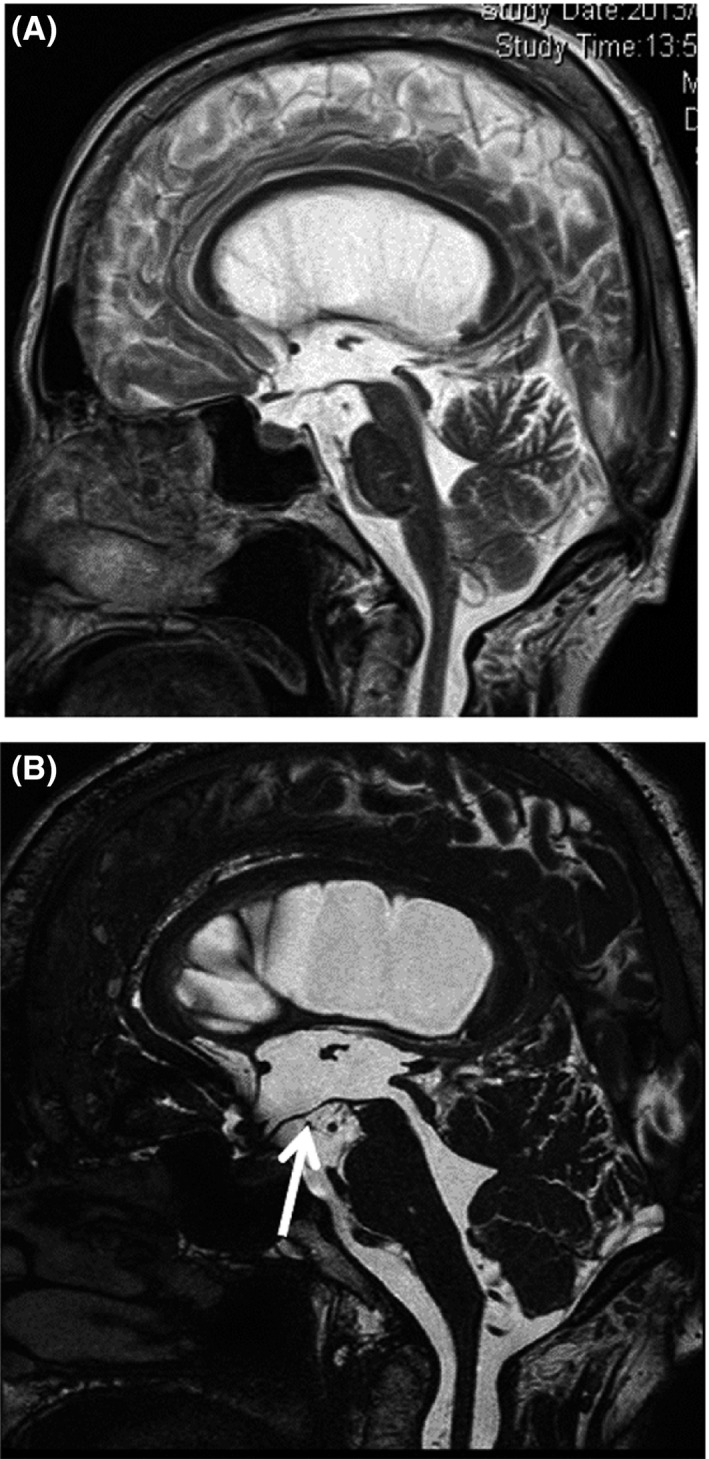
(A) Sagittal T2‐weighted magnetic resonance (MR) image 2 years before admission showing enlargement of the lateral, third, and fourth ventricles. (B) Sagittal T2‐weighted MR image on admission showing slight ballooning of the third ventricle floor (arrow), but no obvious obstruction.
